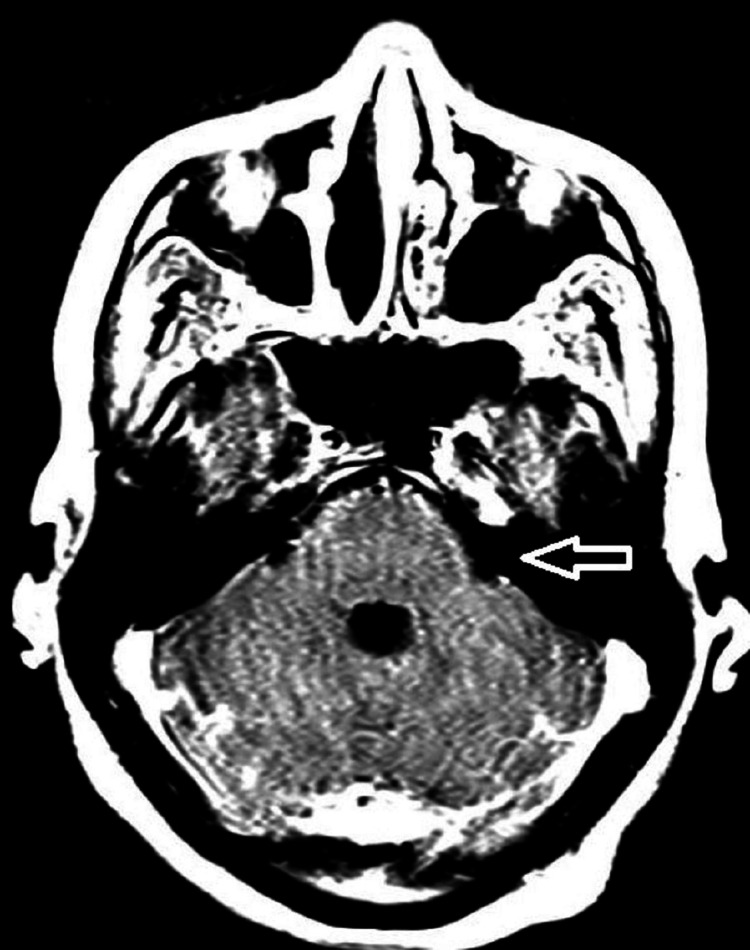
Post-contrast FLAIR imaging with the arrow showing equivocal contrast with mild enhancement. FLAIR: Fluid-attenuated inversion recovery
